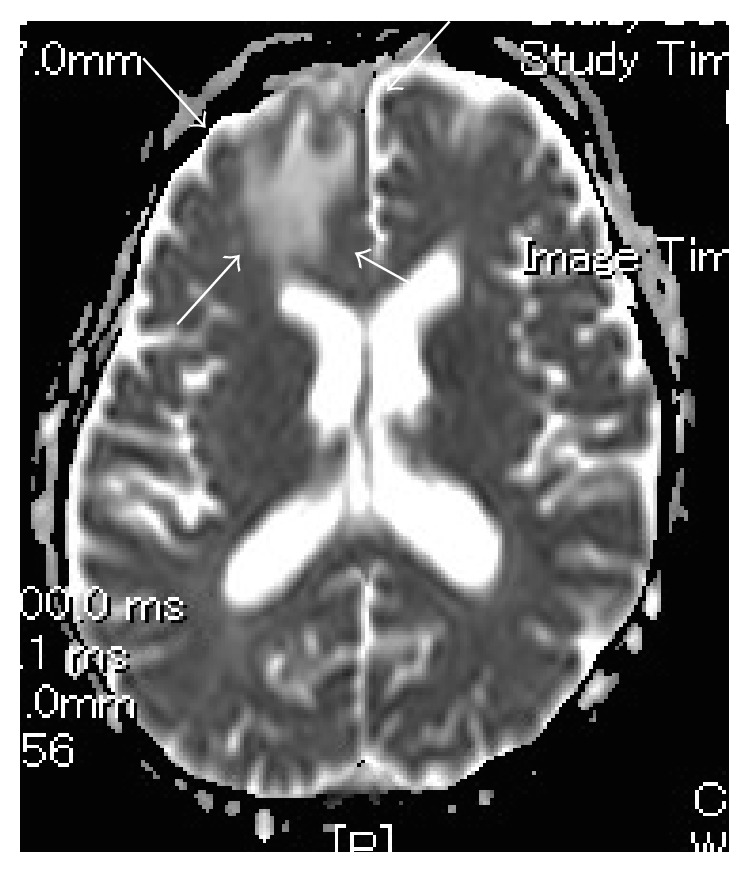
MR imaging (DWI) showing low apparent diffusion coefficient (ADC).
